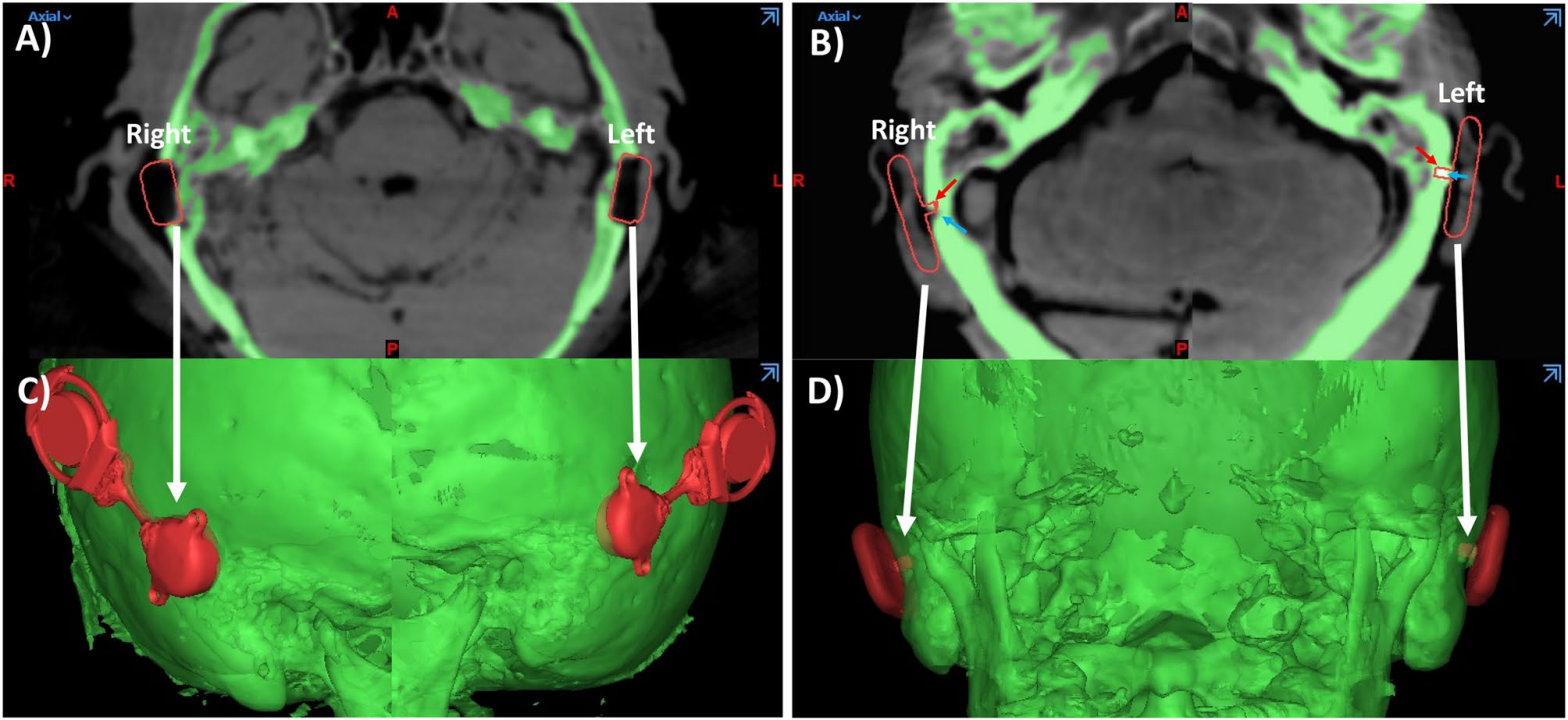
Post-operative CT scans (A) BB-FMT and (B) O-OSI following AR projector guided surgery with paired pre-operative planning rendering seen in (C) and (D). In images (A) and (B), there is the pre-operative planning outline superimposed. The blue arrow denotes post- operative placement whereas the red arrow denotes pre-operative planning.
